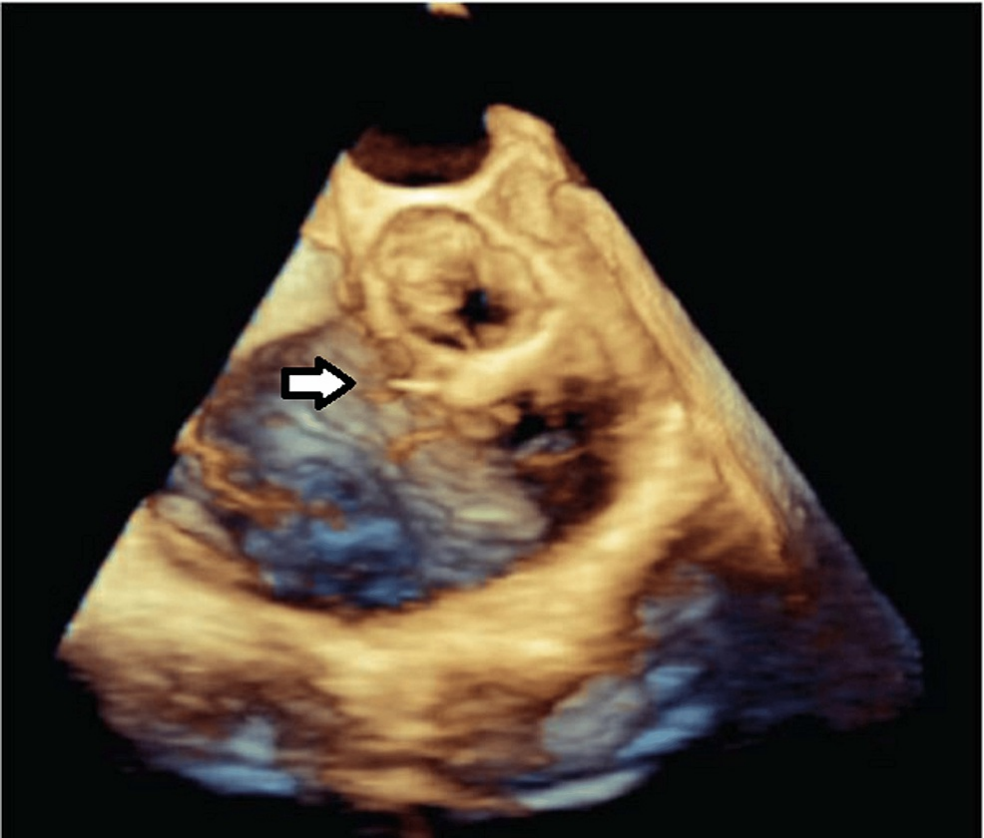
Transesophageal Echocardiogram (TEE). Mid-esophageal TEE 3D still frame showing the aortic valve and root, the tricuspid valve leaflets, and the SOVAR between the non-coronary sinus of the aortic root and the right atrium, providing a 3D view of the defect (the arrow shows the SOVAR).
